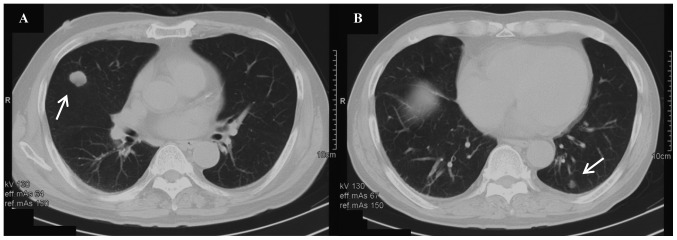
Chest computed tomography revealing two metastatic lung tumors. (A) A tumor measuring 25 mm in diameter is present in the middle lobe of the right lung (arrow); and (B) a tumor measuring 10 mm in diameter is observable in the lower lobe of the left lung (arrow).
